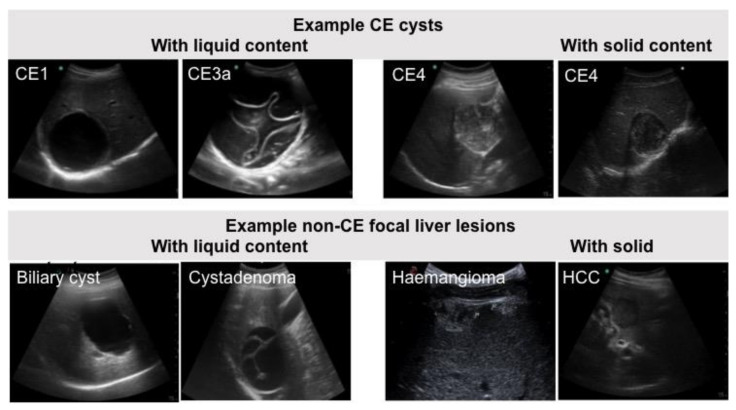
Example ultrasound images of focal liver lesions in differential diagnosis with CE. Top panel: CE cysts in active (CE1) and transitional (CE3a) stages, having a liquid content, and inactive (CE4) stage with solid appearance. Lower panel: focal liver lesions of other etiology with liquid (biliary cyst and cystadenoma) and solid (atypical haemangioma) appearance. HCC: hepatocellular carcinoma.
